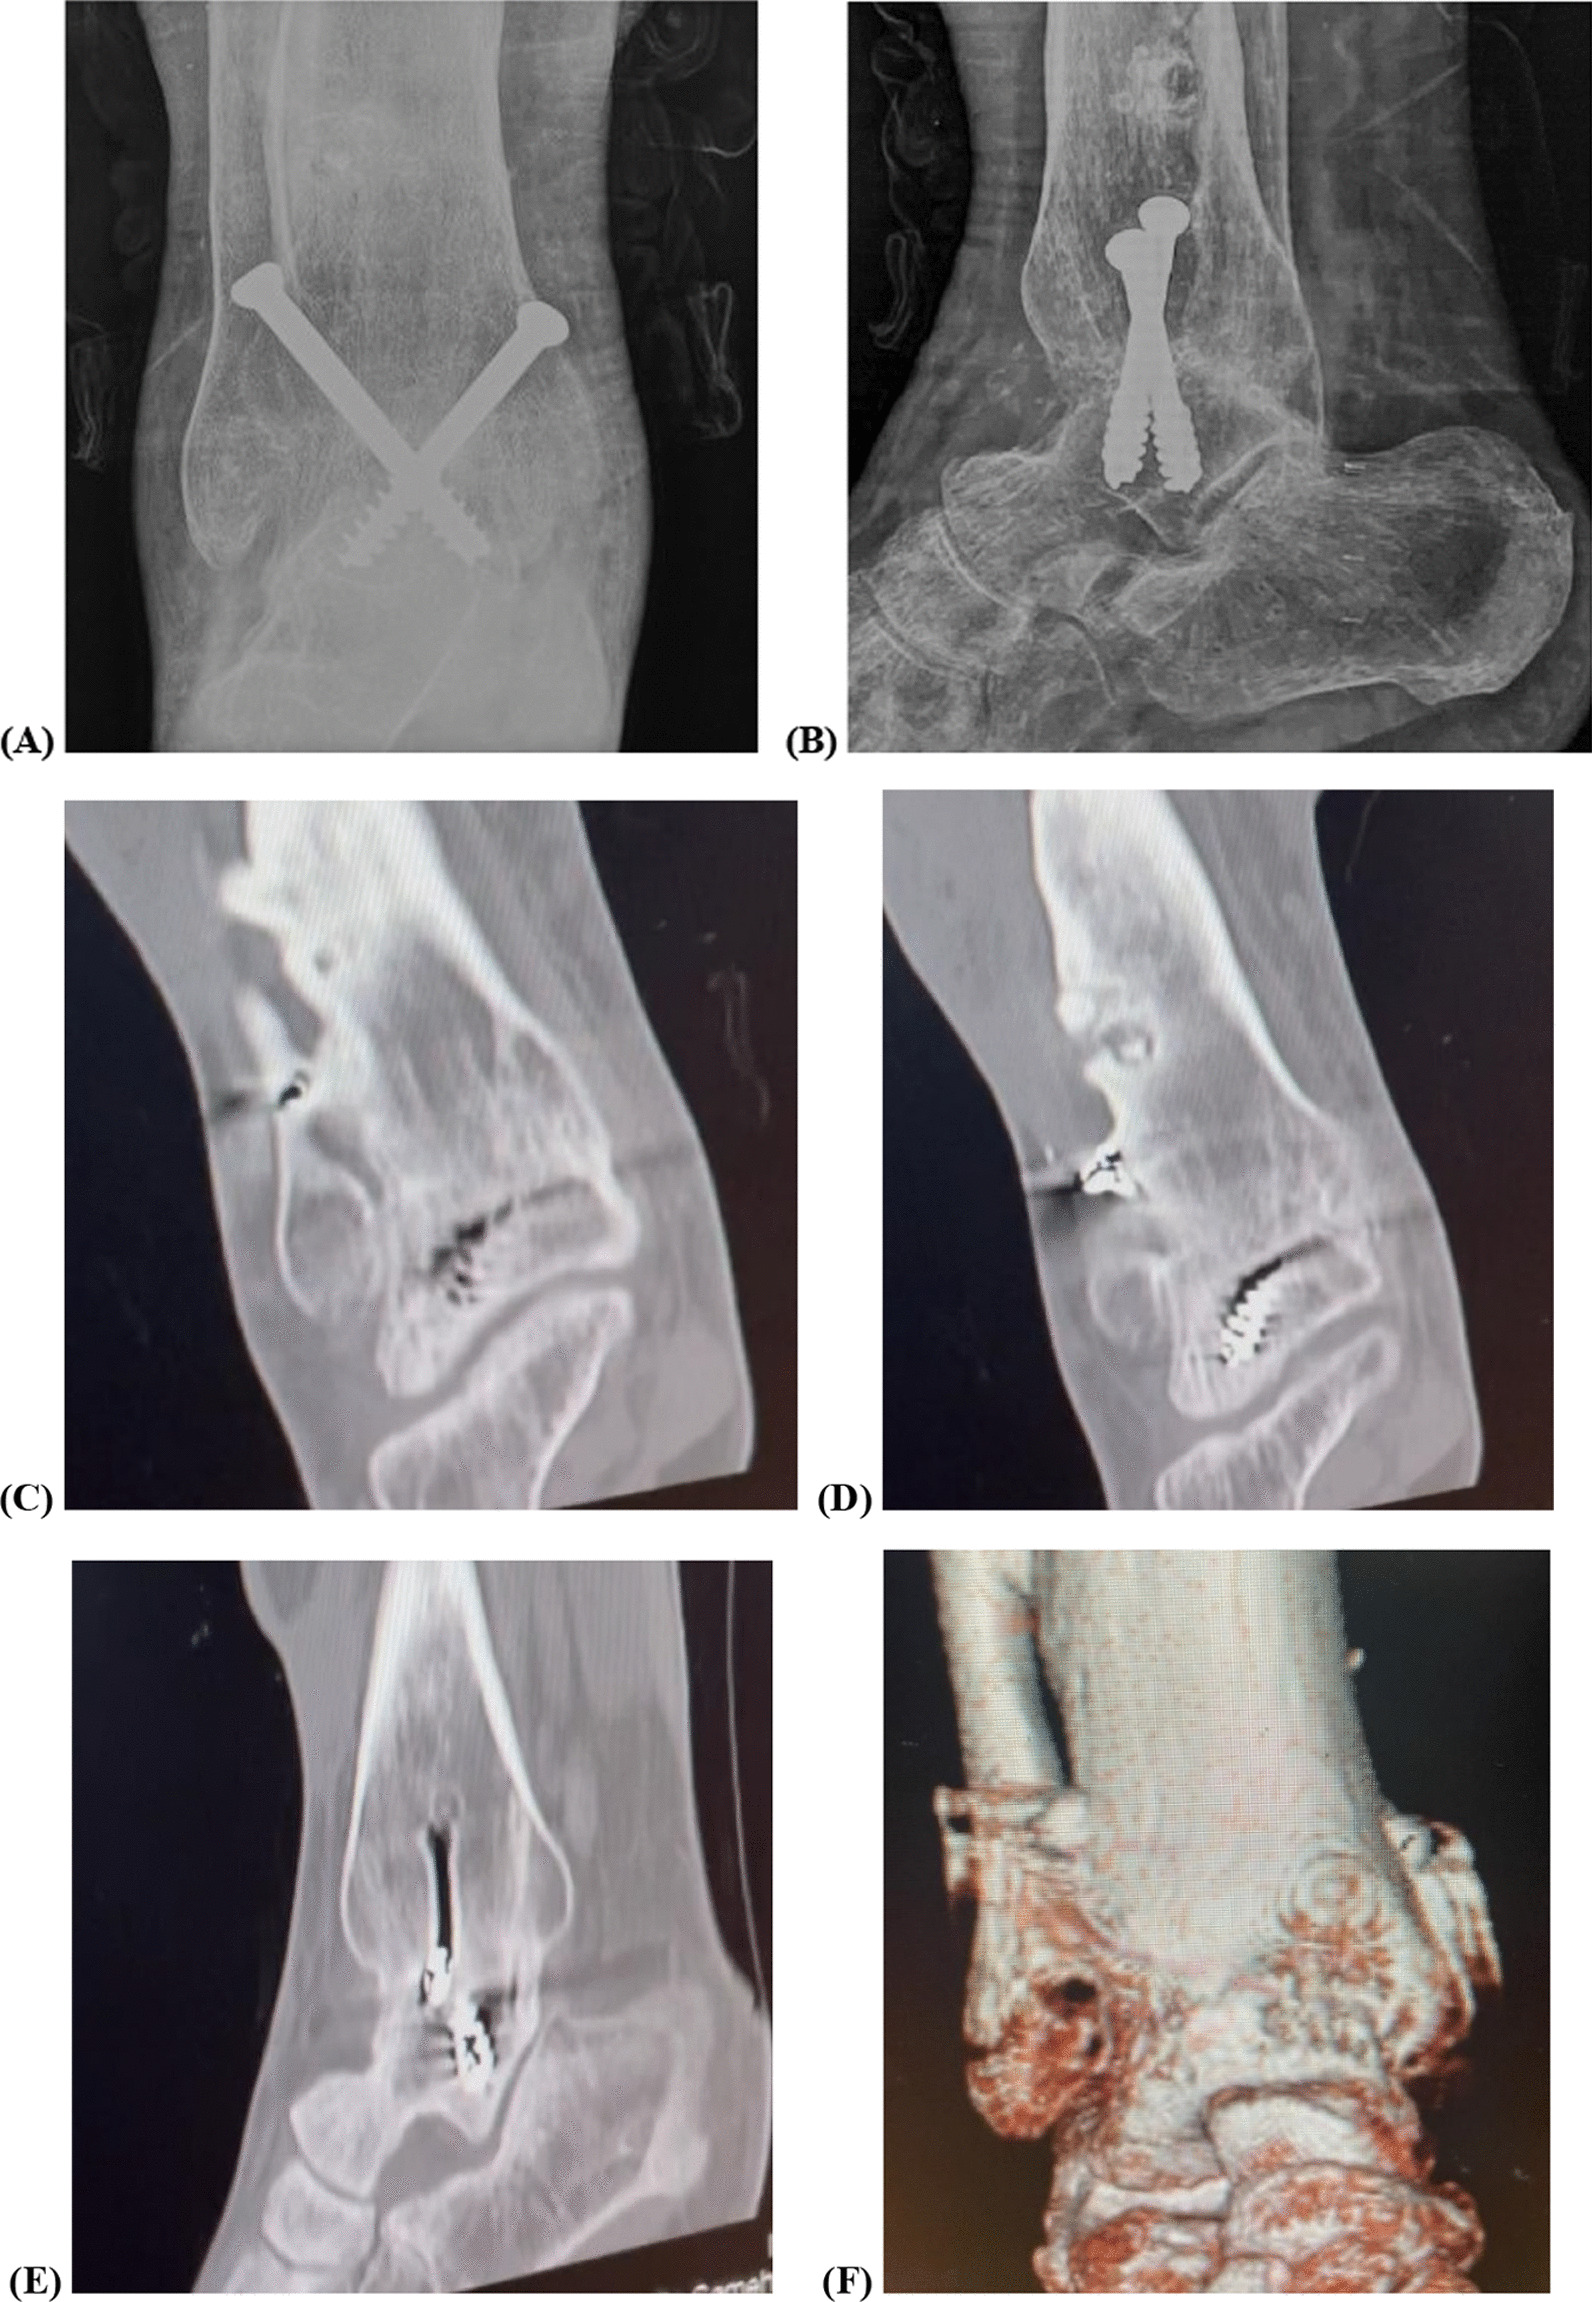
Plain radiographs show radiographic union at 5 months postoperatively in the anteroposterior (a) and lateral views (b), and the CT images demonstrate full consolidation at 9 months (c–f)
